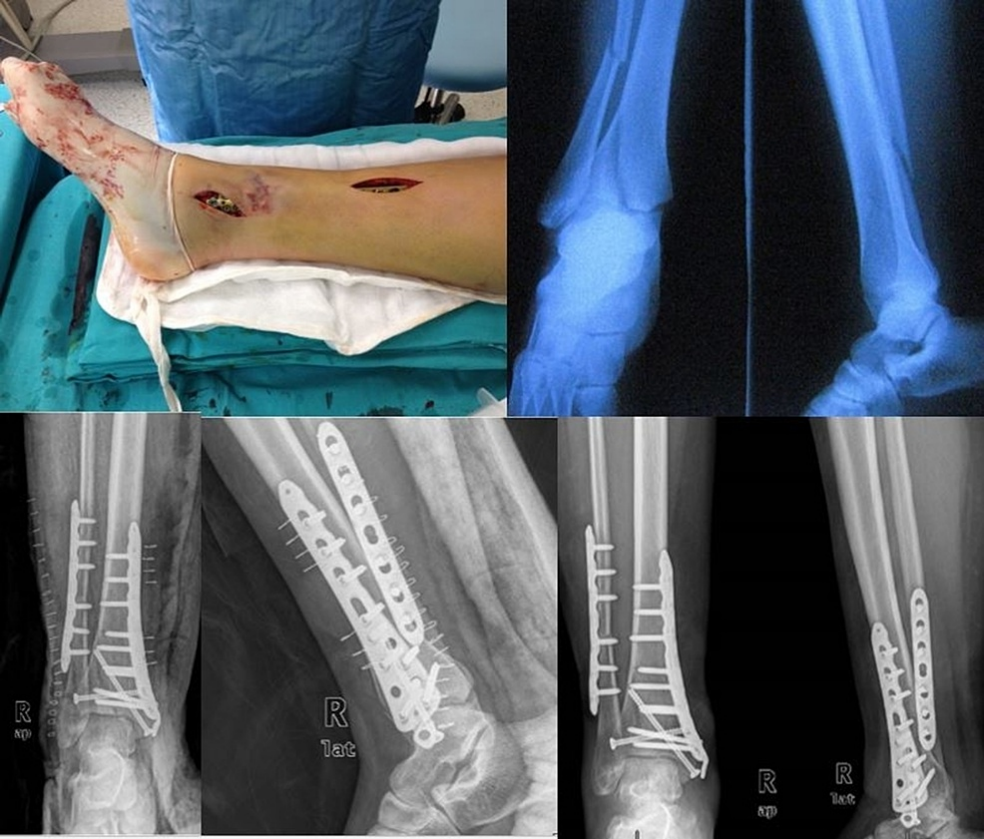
Minimally invasive plating Distal medial anatomical plate placement with a minimally invasive technique. Preoperative and early postoperative X-rays. Cannulated screws were used for joint surface restoration. Second-year control after the operation.
